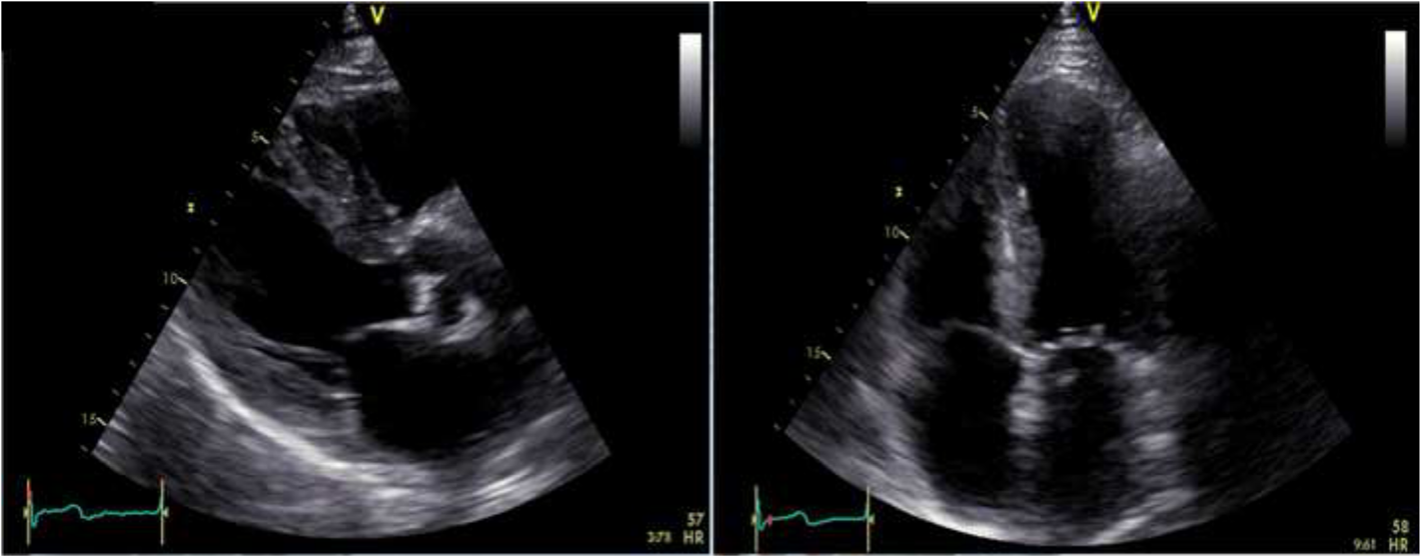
Parasternal long axis (*left*) and apical four-chamber (*right*) views echocardiography images. Videos are available as Additional files 1 and 2
